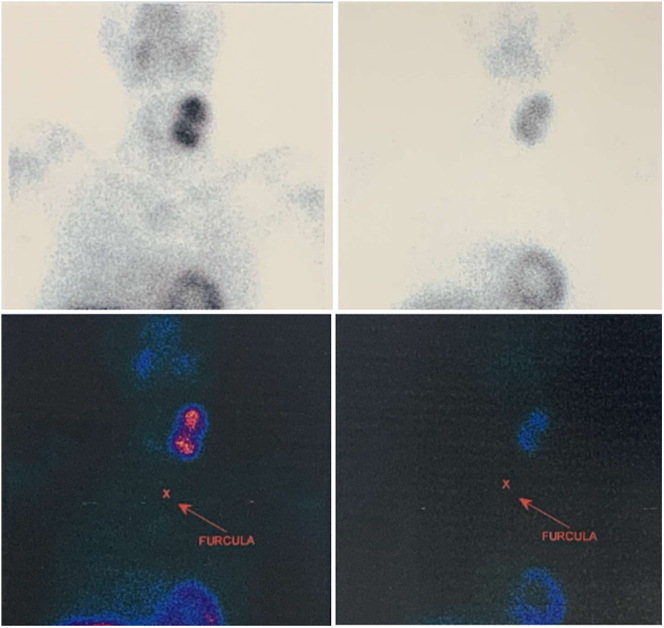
Parathyroid scintigraphy with SESTAMIB with a large area of tracer uptake in the projection of the left lobe of the thyroid (on the left), with significant retention in the late images (on the right).
